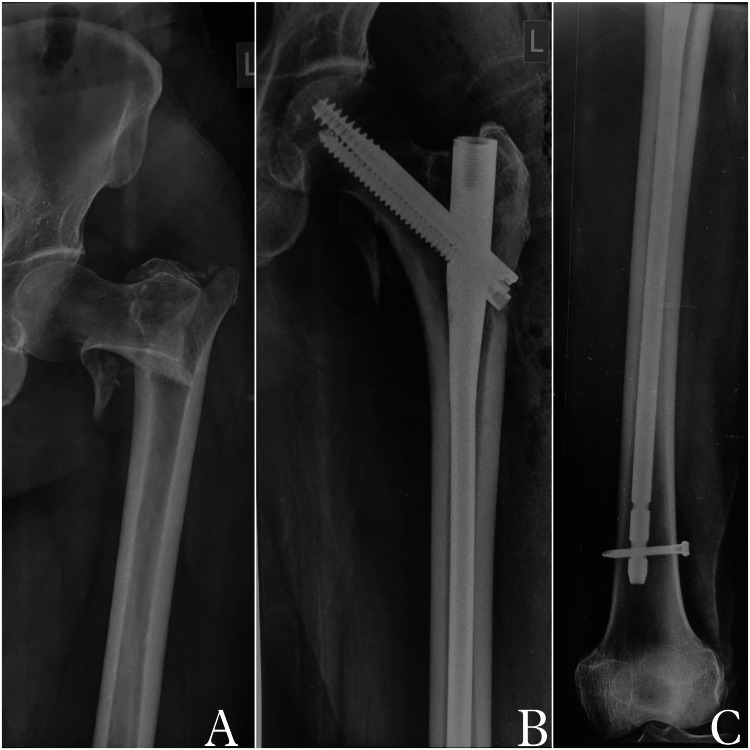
Intertrochanteric fracture fixation using long InterTAN nail (A) Preoperative X-ray showing left intertrochanteric fracture. (B) Two-year follow-up radiograph showing united fracture and long InterTAN nail in situ. (C) Distal locking of InterTan nail seen.
